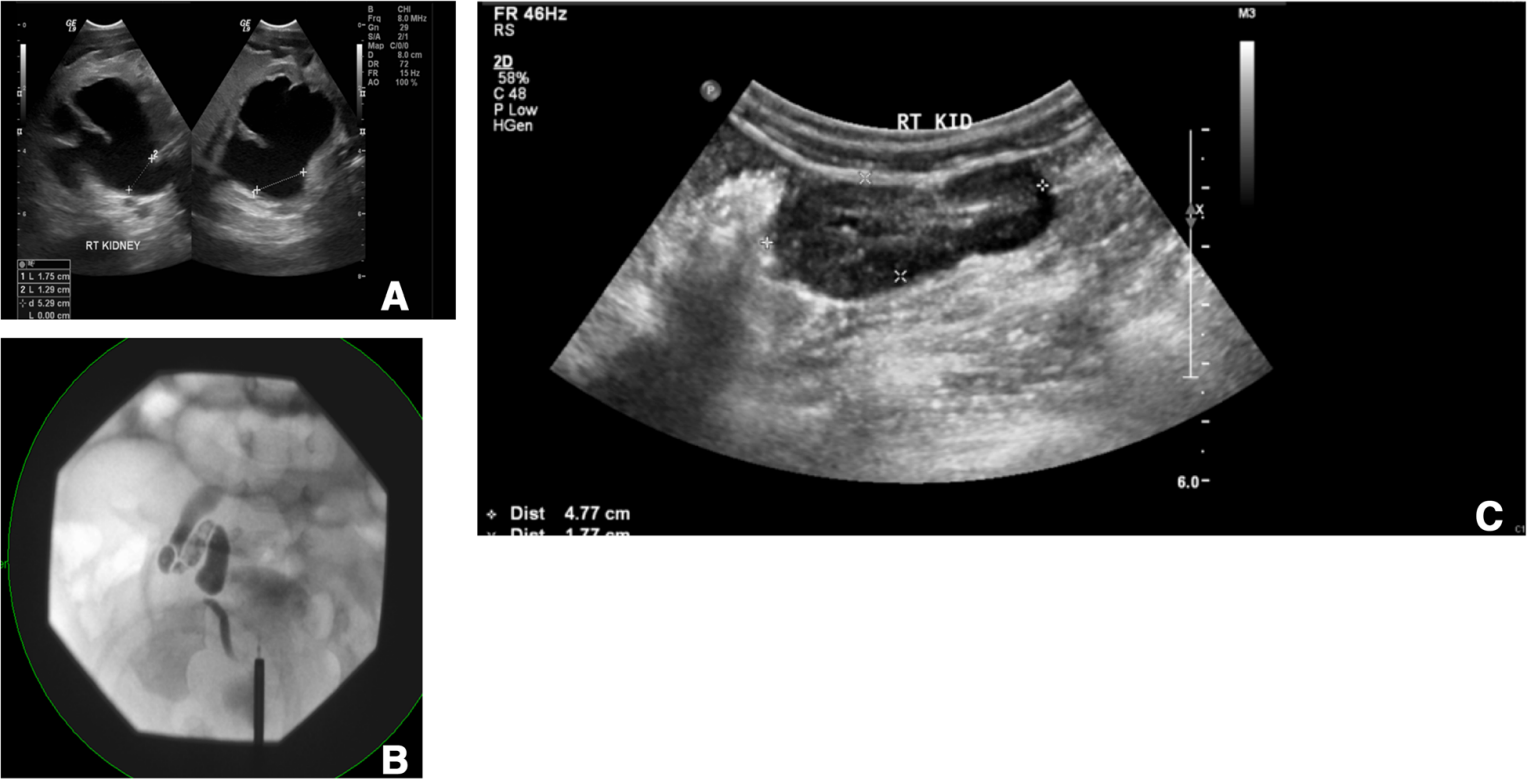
(a) Right renal ultrasound showed SFU grade 4 hydronephrosis. (b)Intraoperative retrograde pyelography showed right ureteral stricture at the level of the lower sacroiliac joint. (c)Renal ultrasound at 18 months postoperatively
